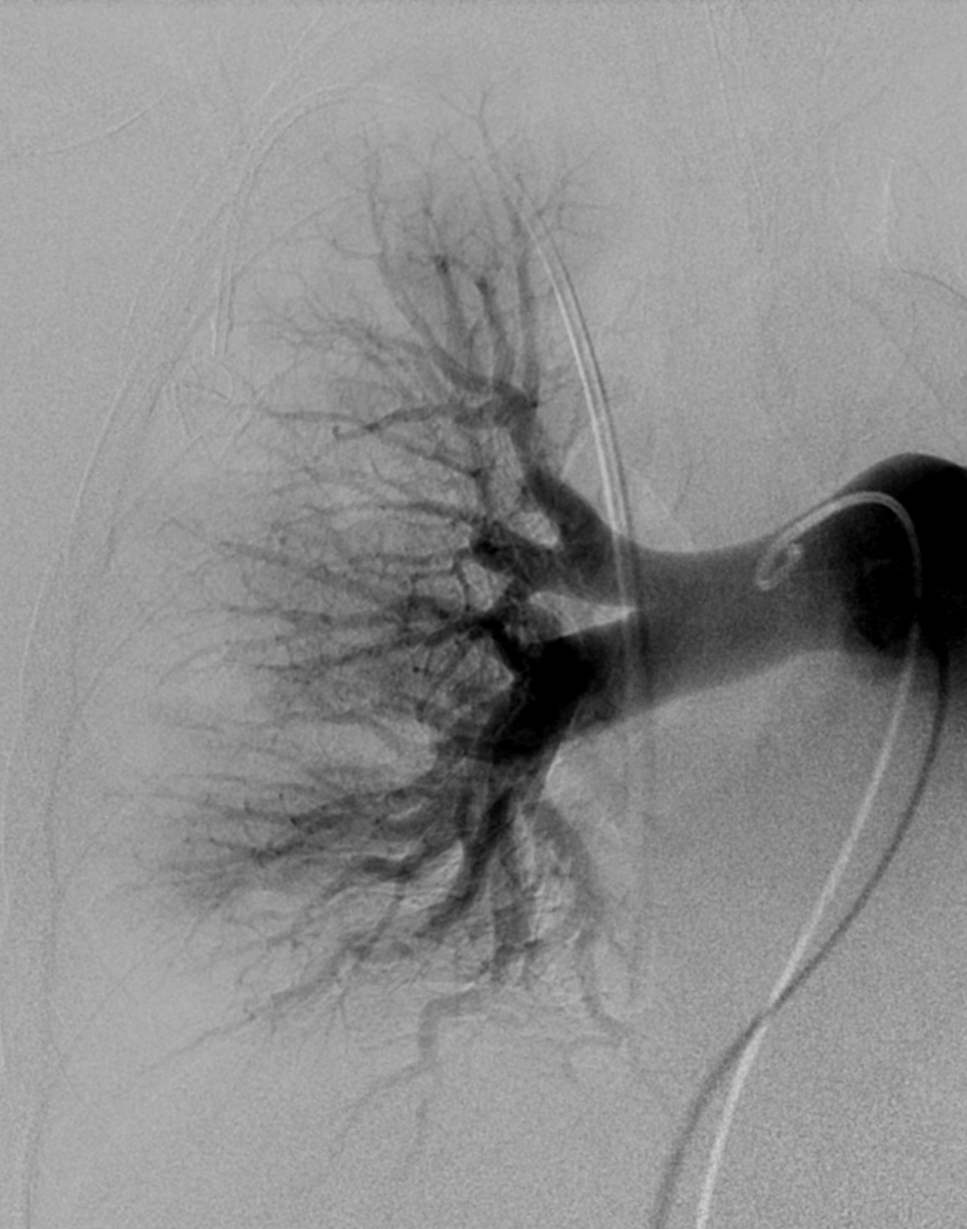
Right Lung Post-Mechanical Embolectomy CT Angiography
